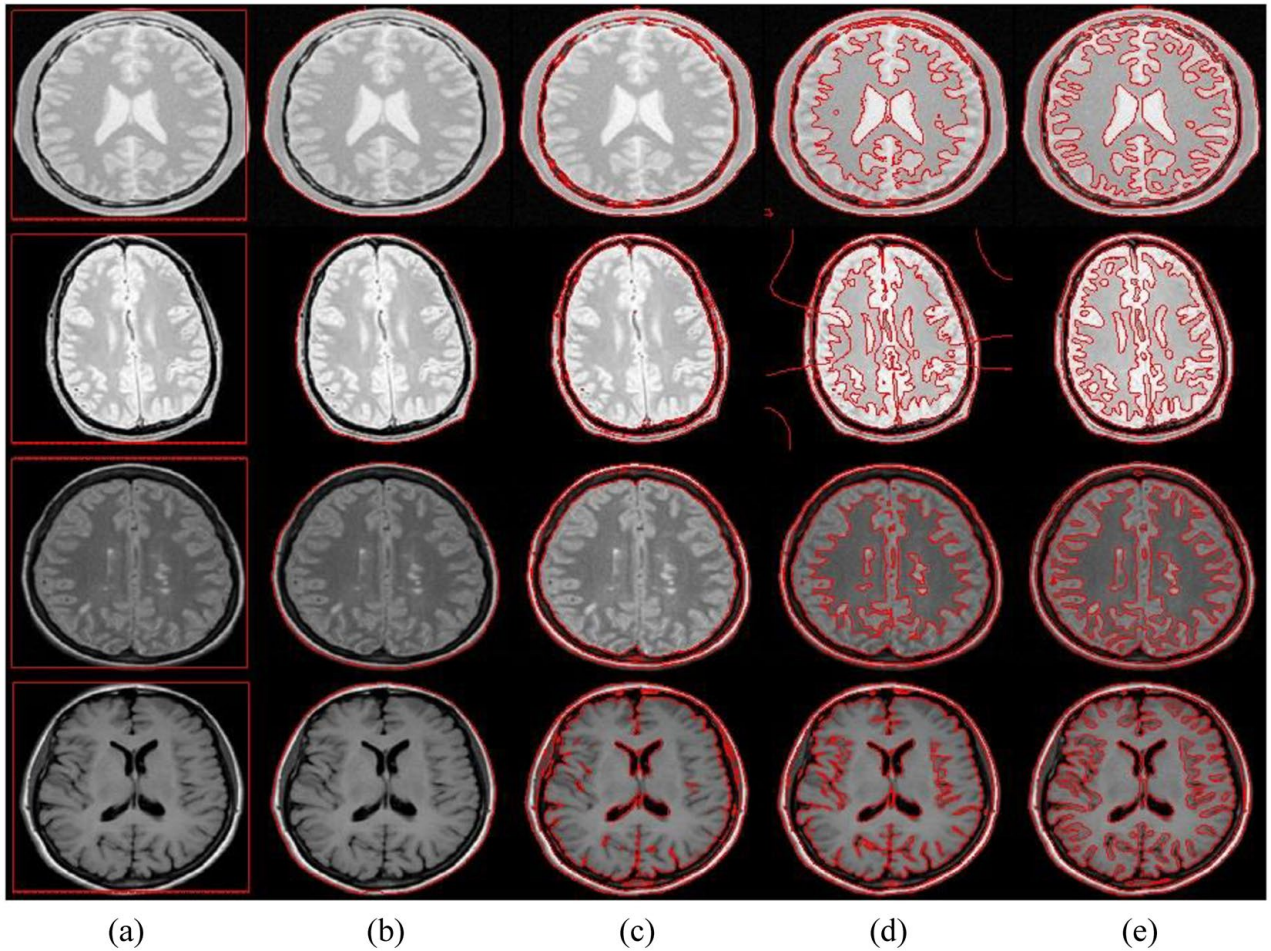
Brain MR image segmentation comparison with the state-of-the-art. (a) Initial contour, (b) DRLS, (c) CV, (d) RSF, (e) Proposed method.
